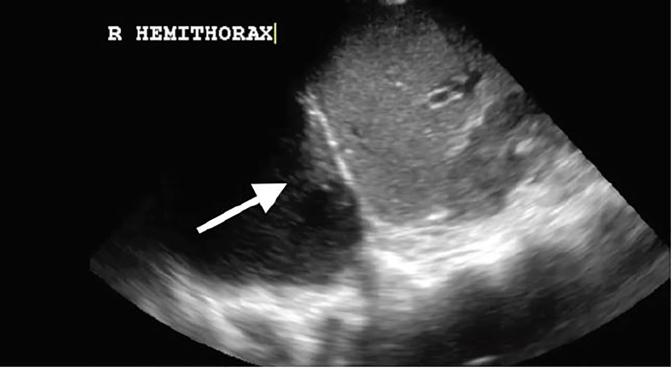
Thoracic point-of-care ultrasound using 4-megahertz curvilinear probe, demonstrating an empyema in a coronal view (arrow). See video.
